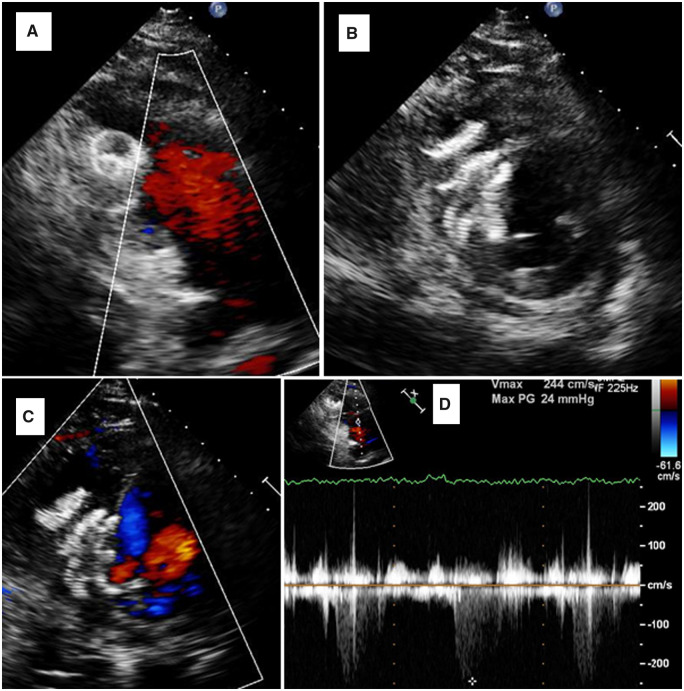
(A) Transthoracic echocardiogram at follow-up, right ventricular inflow view showing the right ventricular disc of the 18-mm device. (*B*) Transthoracic echocardiogram short-axis view showing both devices, with the aneurysmal cavity closed. (*C*) No more colour flow to aneurysm or right ventricle. (*D*) Estimated systolic pulmonary artery pressure of 35 mmHg.
